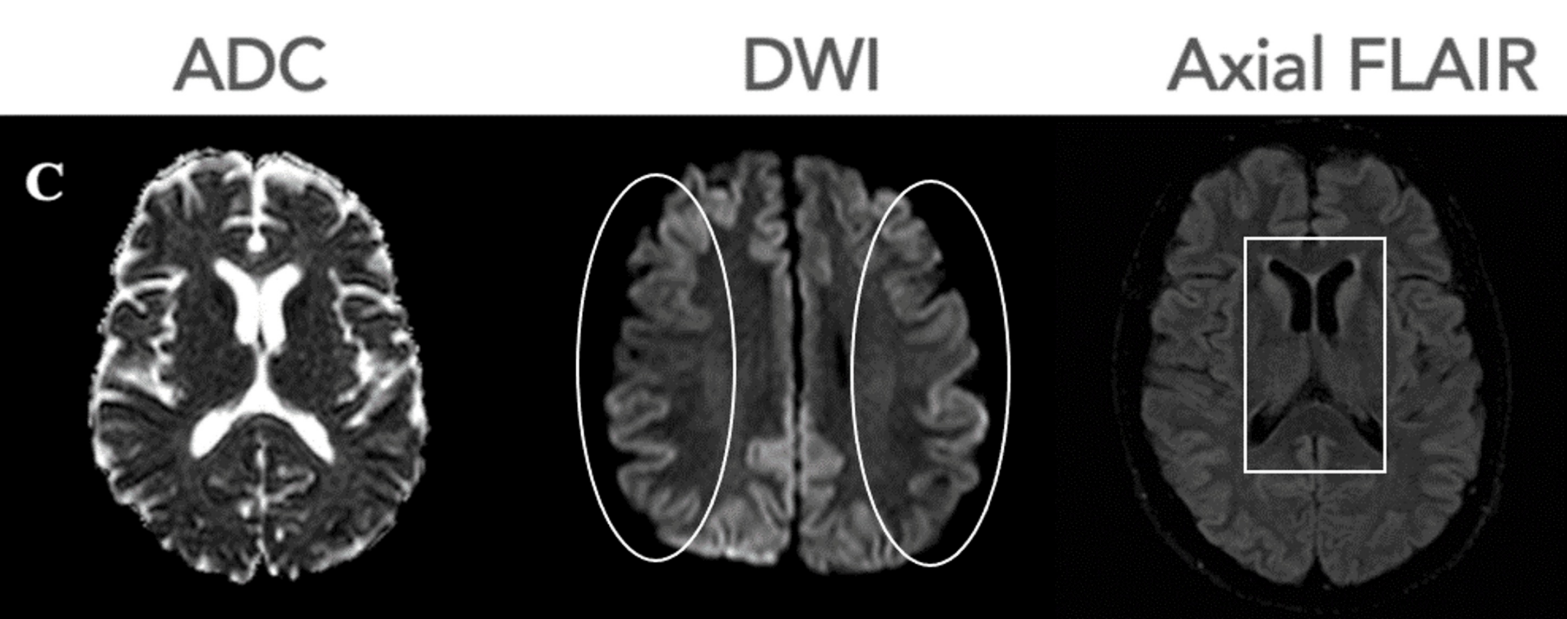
A third brain MRI was obtained on October 11, 2022. The entire cortex of both cerebral hemispheres showed intermediate to high T2/FLAIR signal intensity in the frontal, parietal, and temporal lobes, with similar changes now evident in the caudate, putamina, and thalami as noted on the axial FLAIR (square). Signal hyperintensity of the cortical gyri (cortical ribbon sign), typical of Creutzfeldt-Jakob disease, is now more evident on DWI (circles). ADC: apparent diffusion coefficient map; DWI: diffusion-weighted image; FLAIR: fluid attenuated inversion recovery
